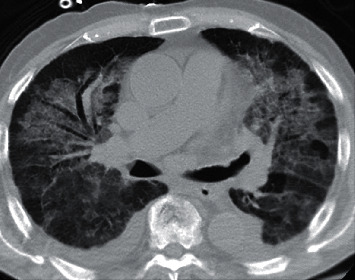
CT chest demonstrating diffuse ground glass opacities bilaterally, central predominant, no focal infiltrates.
